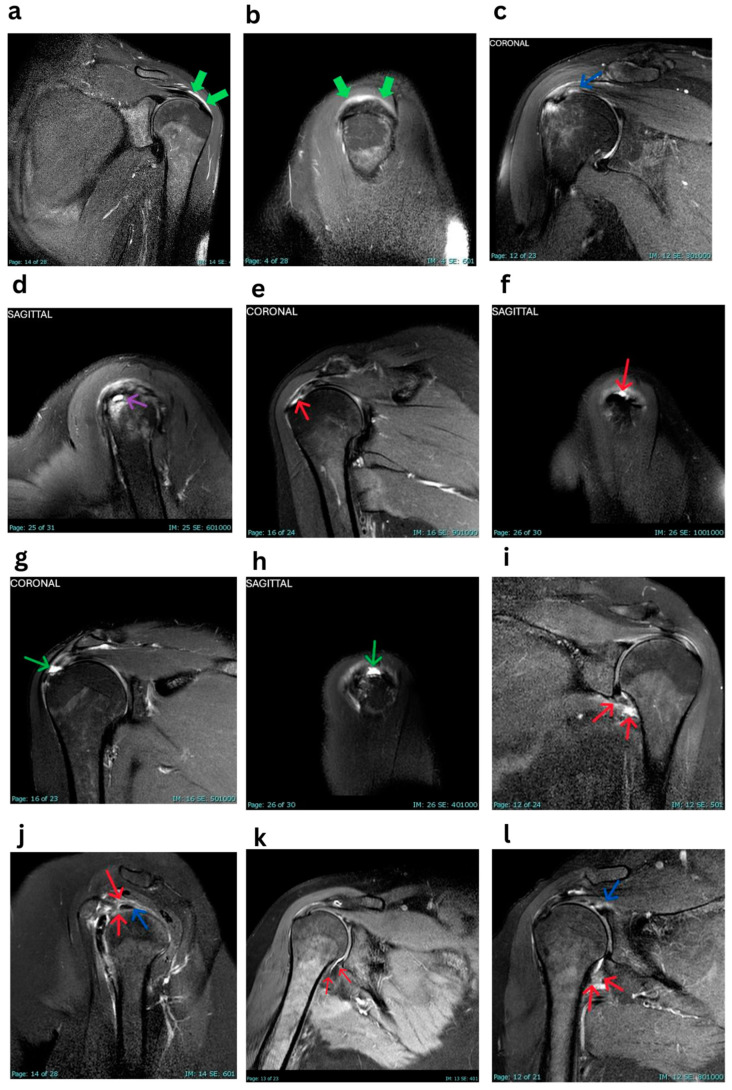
MRI findings. Thickened edematous subacromial-subdeltoid bursa in (a) coronal and (b) sagittal T2-SPAIR MR images (thick green arrows). Rotator cuff disease in (c) coronal and (d) sagittal (supraspinatus tendinopathy, blue arrow in (c), and purple arrow in (d)), and partial thickness tear in (e) coronal and (f) sagittal (red arrows). Full thickness tear in (g) coronal and (h) sagittal (thin green arrows). Thickened edematous glenohumeral joint capsule at (i) the axillary pouch (red arrows) and (j) rotator cuff interval (red arrows), indicating adhesive capsulitis. Note the intra-articular portion of the long head of the biceps tendon in (j) (blue arrow) and how it is clearly visible as a signal void structure in a background of the edematous capsule of the high T2 signal. Interval development of adhesive capsulitis after two years and eight months at the (k) initial and (l) follow-up MRI studies. In addition to edema at the axillary pouch in the follow-up images (red arrows in (l)), note the edema adjacent to the superior labrum (blue arrow in (l)) where the capsule also attaches.
